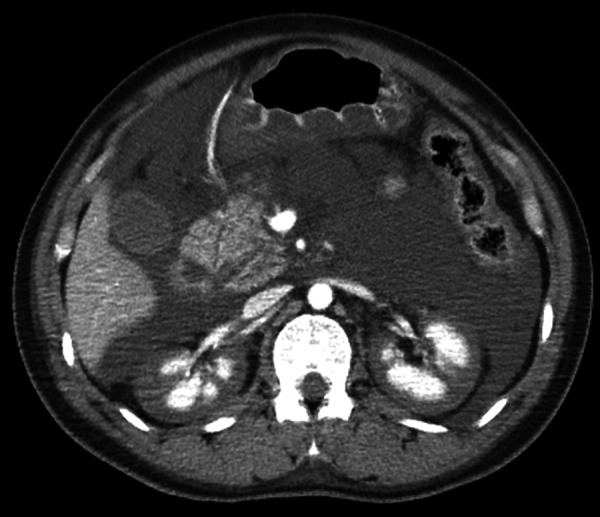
Abdominal contrast-enhanced computed tomography findings. The renal cortex displays a non-enhancing pattern, while the medulla demonstrates enhancement, which is indicative of the reverse rim sign consistent with renal cortical necrosis.
